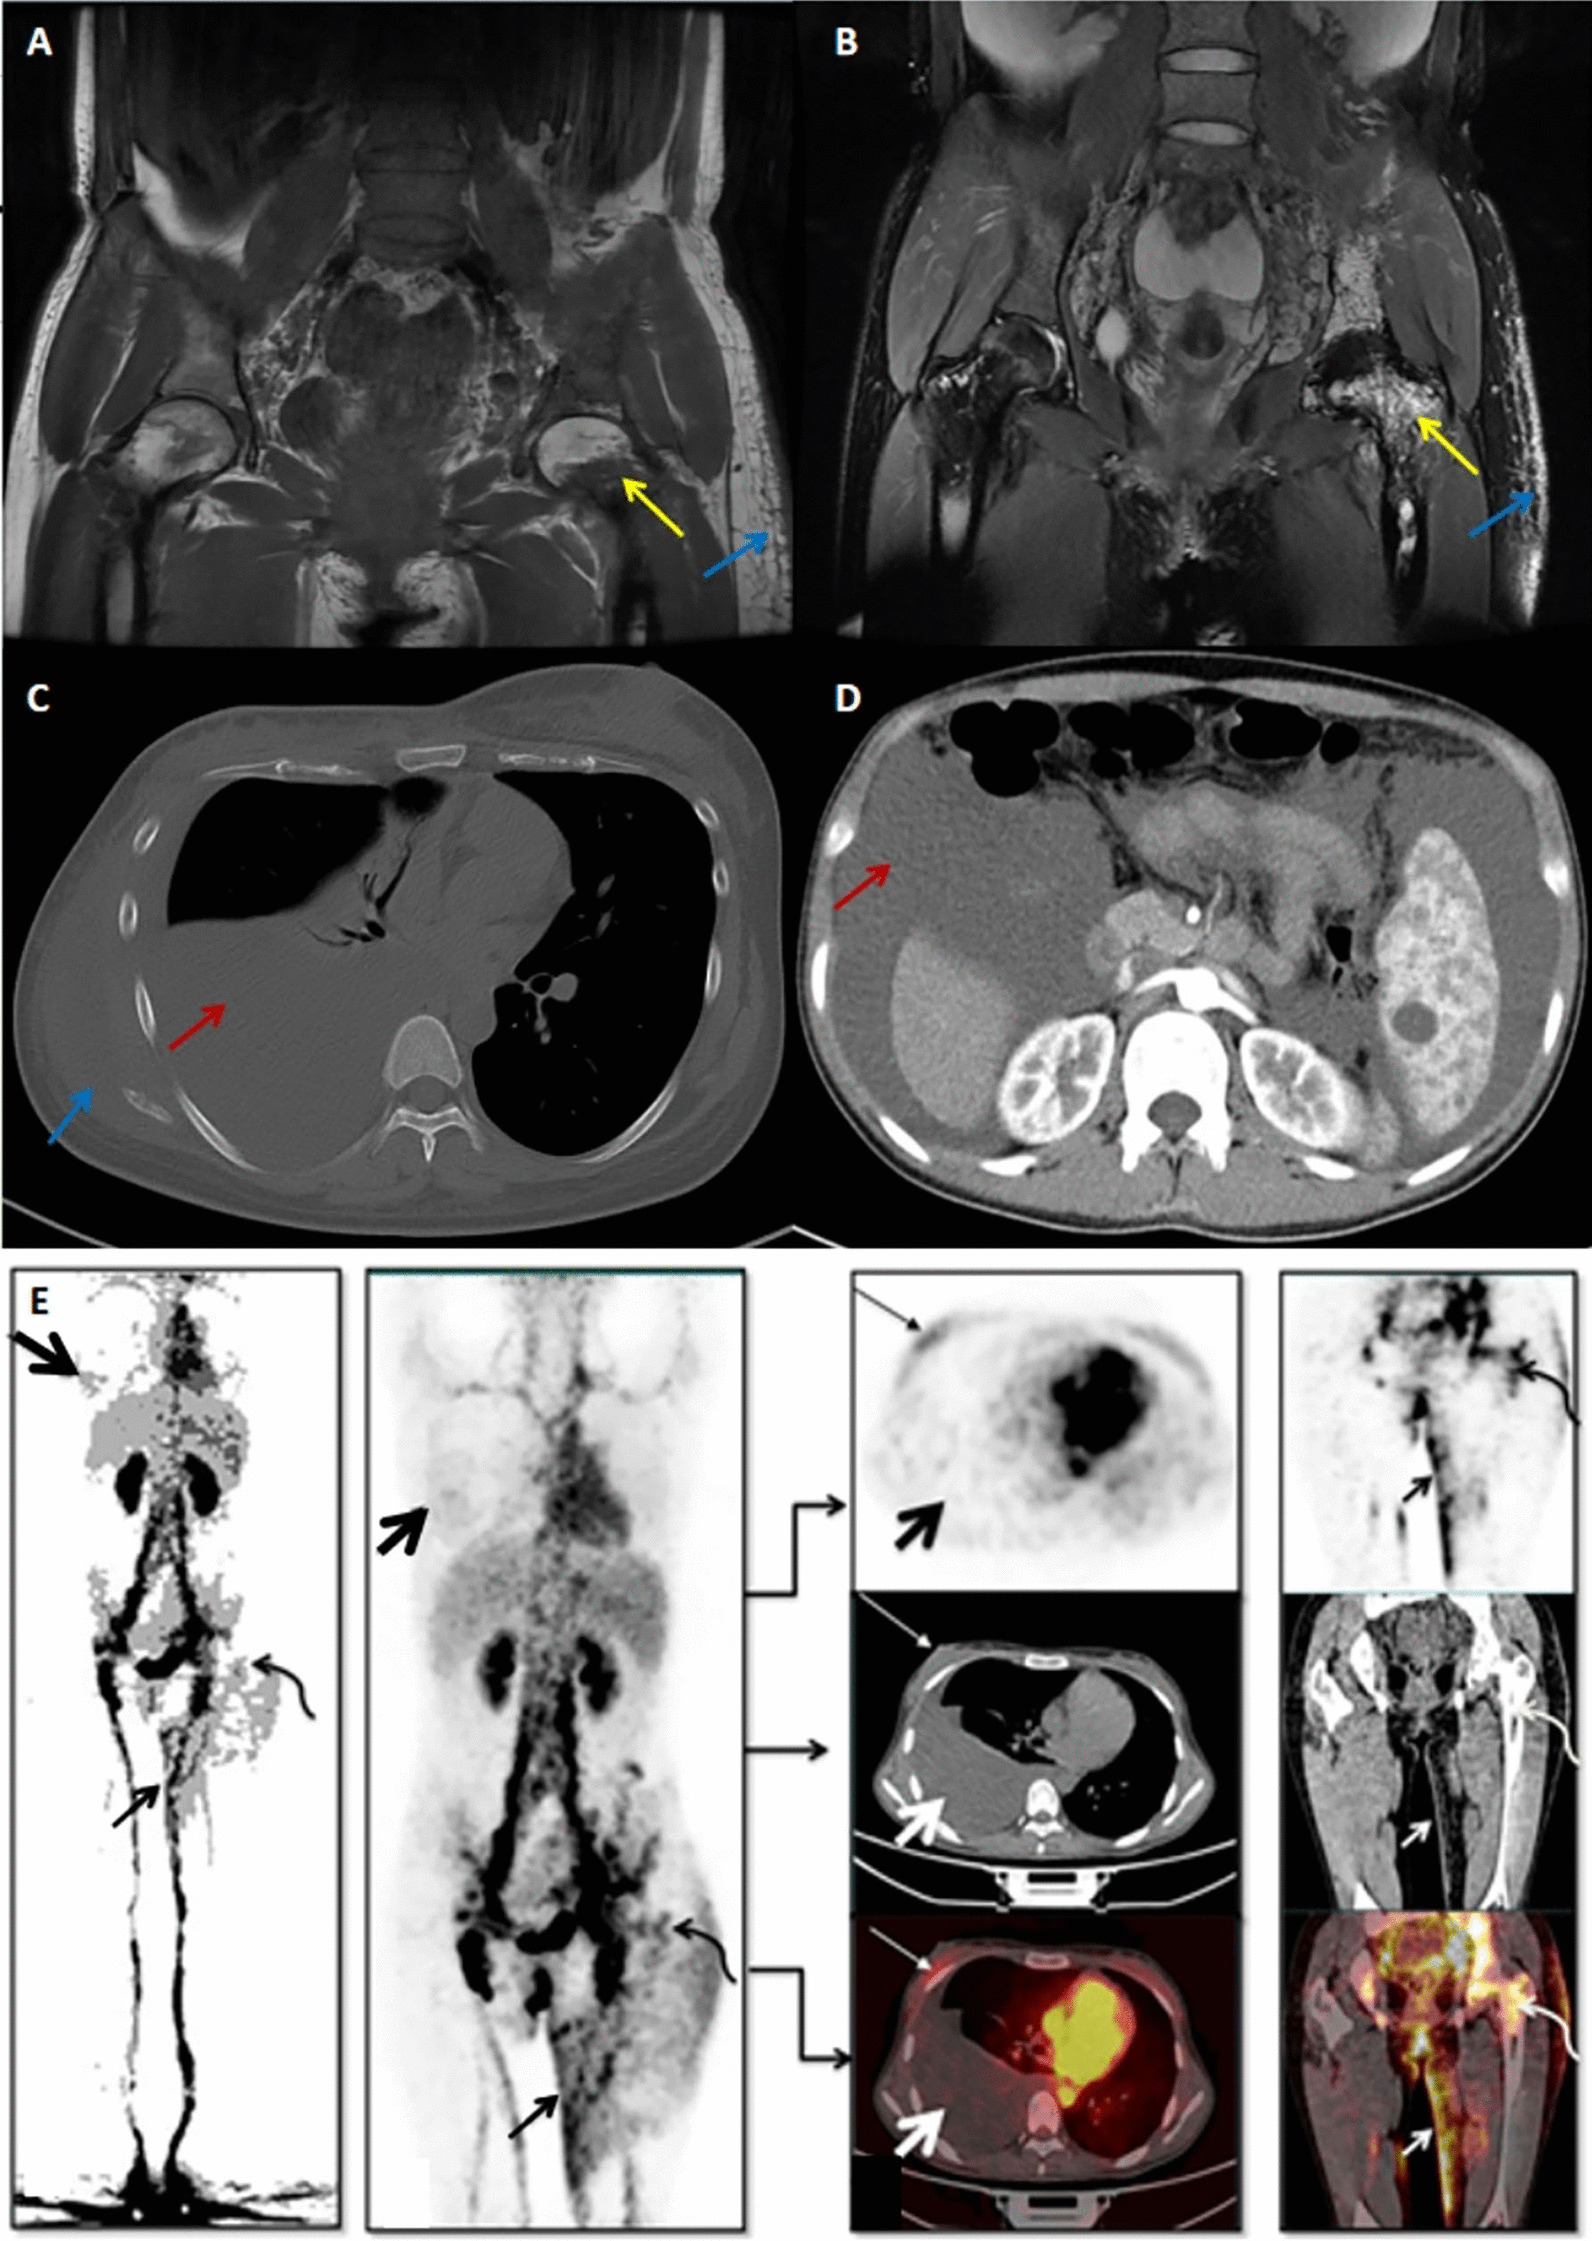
Characteristic imaging findings of GSS. A and B Bone lesion (yellow arrows) of the left femur and the incrassated subcutaneous tissue (blue arrows) of the left hip on MRI T1 and T2 images. C and D Massive pleural and peritoneal effusion, respectively (red arrows), and the incrassated subcutaneous tissue (blue arrows) of the right chest wall on CT images. E Increased uptake of ^68^Ga-NEB in femur (thin, curved arrow), pleural effusion (thick, straight arrow) and skin (thin, straight arrow) on ^68^Ga-NEB-PET/CT. MRI, Magnetic resonance imaging; CT, computed tomography; ^68^Ga-NEB-PET/CT, ^68^Ga-NOTA-Evans Blue-positron emission tomography/computed tomography
